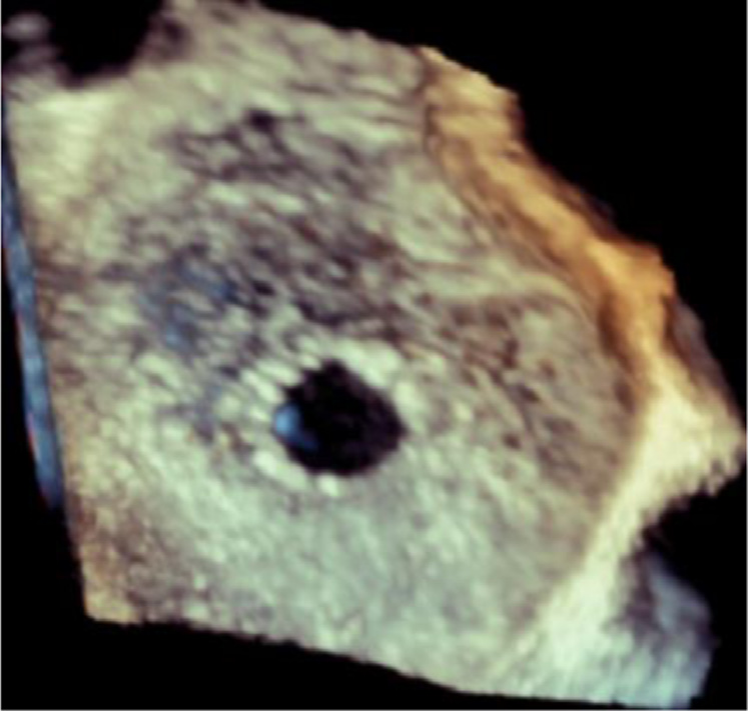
Representative images of 3D transesophageal echocardiographic assessment of interatrial shunt immediately following shunt creation in a human subject.
